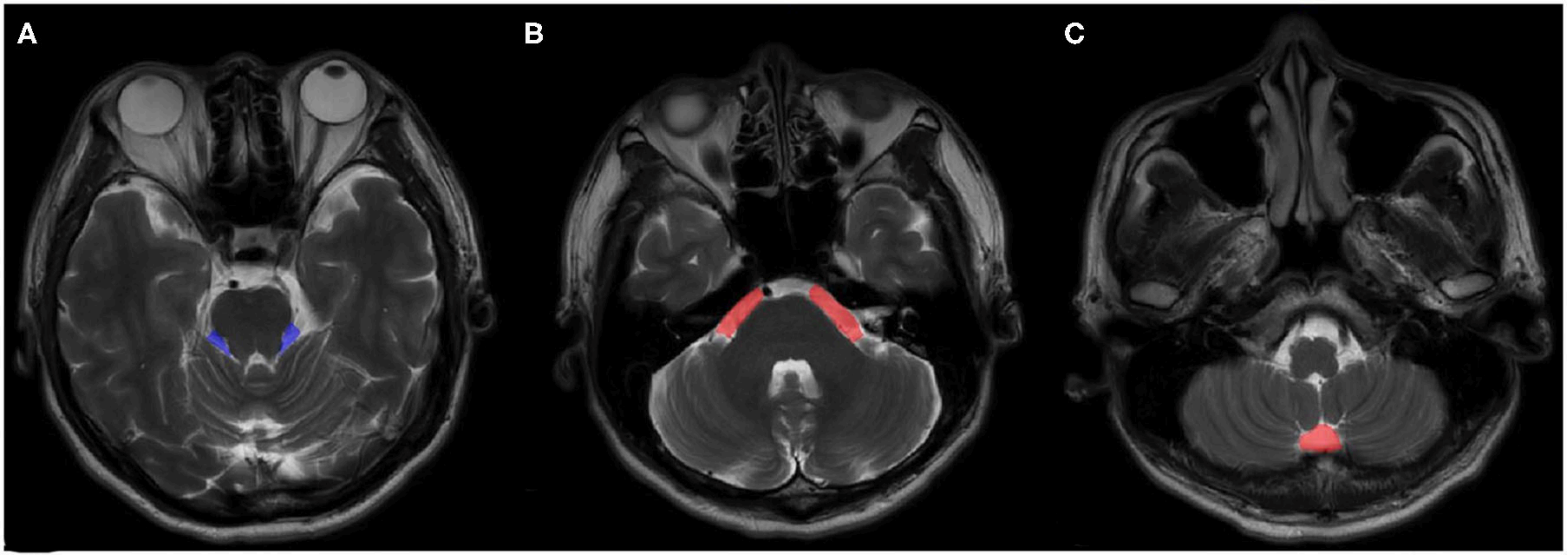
An example of ROI views on MRI. (A) cerebellopontine cisterns, (B) precerebellar cistern, (C) prespinal cistern.
